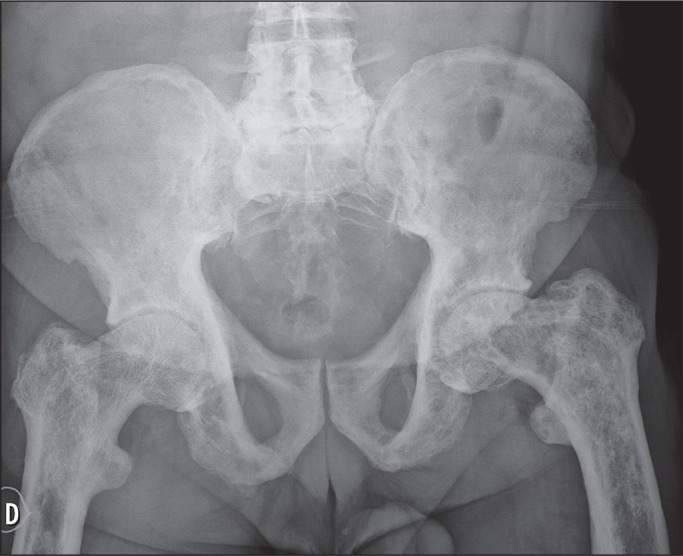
Anteroposterior X-ray of the pelvis of a patient with a confirmed
